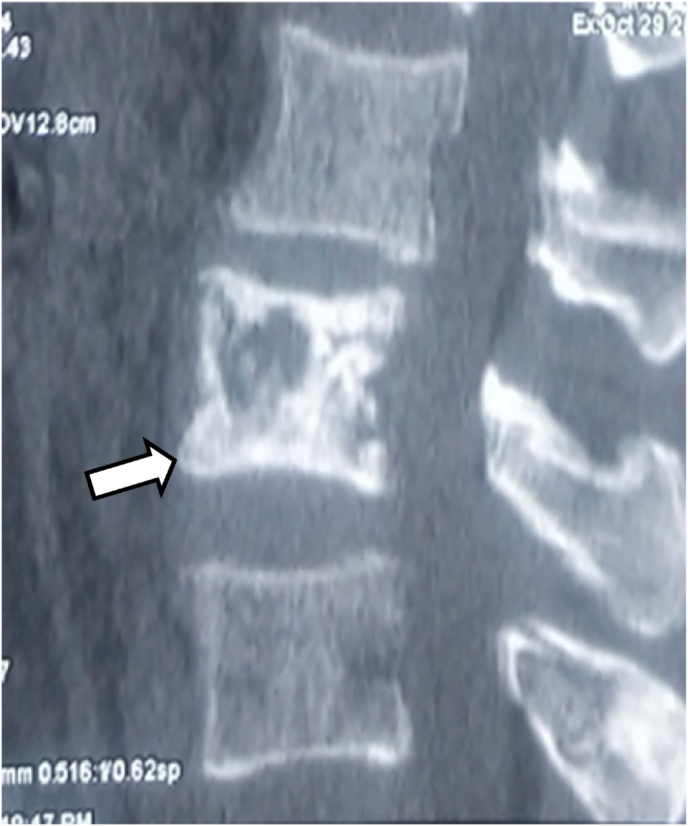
Sagittal CT shows diffuse sclerosis (white arrow) of the involved vertebra with ill-defined lytic areas.
